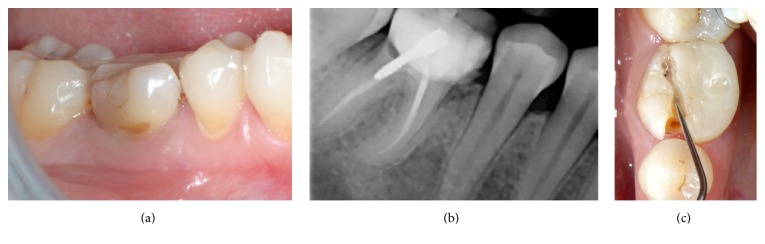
Nonrestorable mandibular first molar. (a) Initial clinical situation; (b) periapical X-ray; (c) occlusal view showing the mesiodistal fracture.
